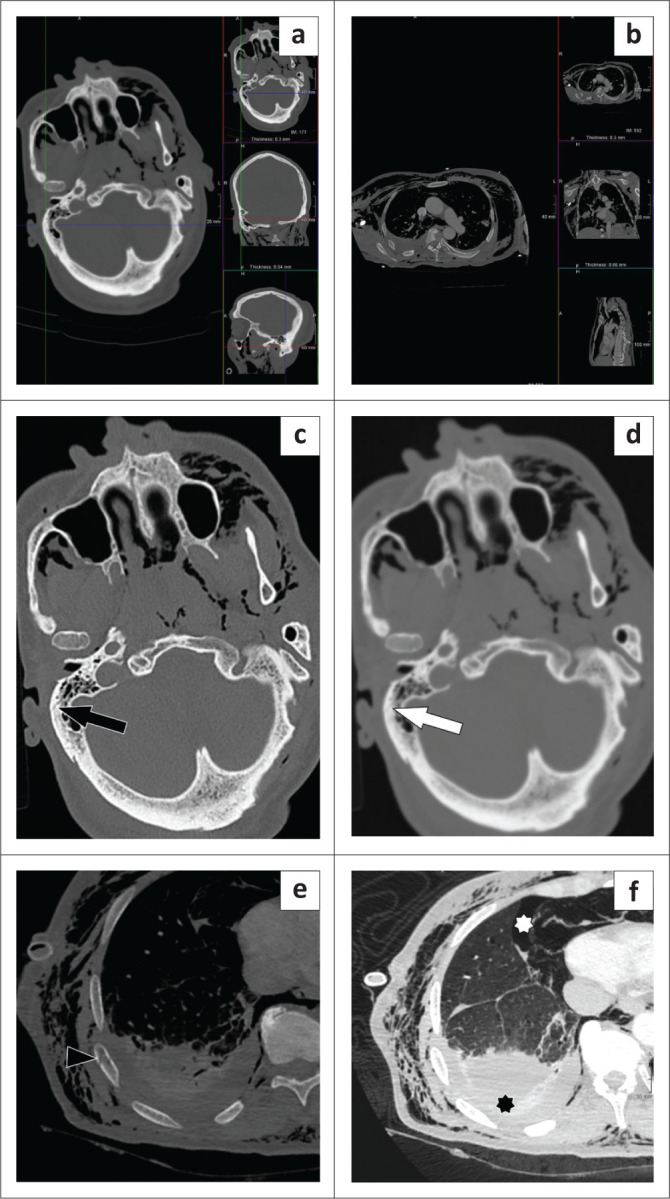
A CT pan-scan showing extensive subcutaneous emphysema of both the head (a) and chest (b). When viewed without straightening the scan using multiplanar reconstruction (c), the right temporal bone otic capsule–sparing fracture is not easy to detect even utilising the bone reconstruction algorithm (black arrow) and nearly invisible (white arrow) on the soft tissue reconstruction algorithm (d). The bone window (e) demonstrates an initially overlooked nondisplaced right 9th rib fracture (black arrowhead). Note the right haemothorax (black star) and pneumothorax (white star) seen on the lung window of the chest CT (f). Also note that the patient was ‘scanned skew’.
